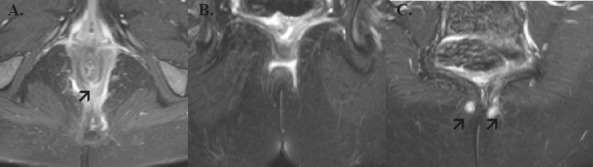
Axial sections of An MRI T2 Weighted image with fat suppression shows a horseshoe fistula tract with internal opening seen at 6 o'clock (shown by an arrow in A) considering the anal canal as a clock face. The tract crosses the midline (seen in B) and has two external openings to the right and left of the natal cleft (shown by the arrow in C)
